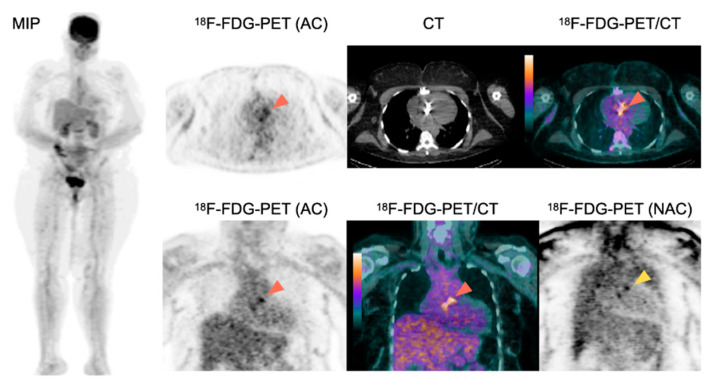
Role of ^18^F-FDG-PET/CT for the diagnosis of PVE.
